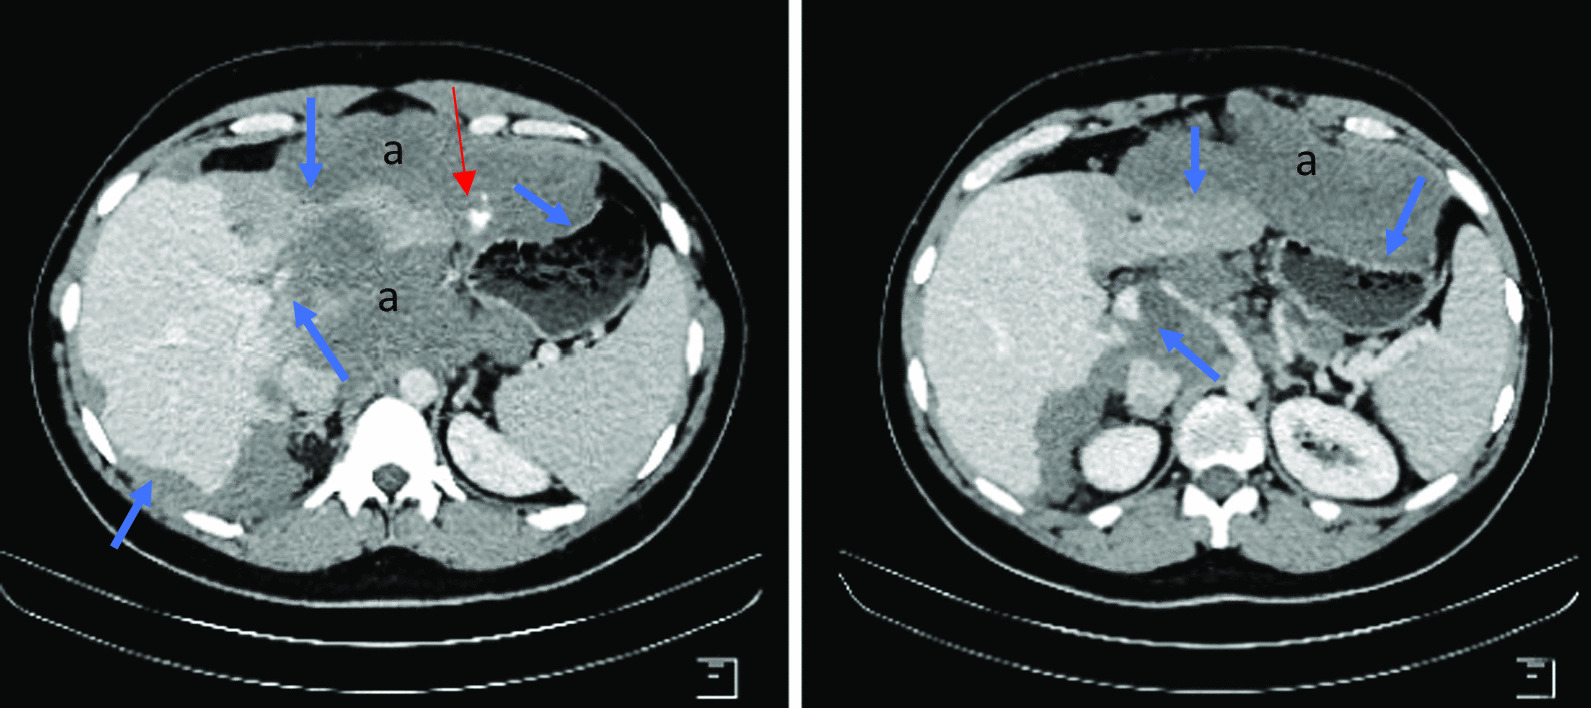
Case 3: axial sections of abdominal CT scan with injection at venous time, showing tissue mass (a) responsible for a mass effect on the stomach and scalloping on the left liver, hepatic hilum, and perihepatically (blue arrows). Red arrow indicates some calcifications inside the tumor
